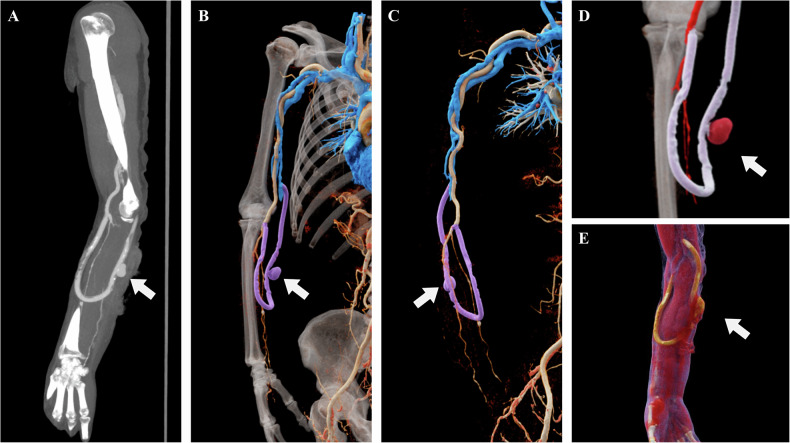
Follow-up images of a 65-year-old male after prosthetic arteriovenous fistula creation. A The CTA-based MIP image reveals an abnormal encapsulated hyperdense shadow surrounding the prosthetic graft, suggestive of hemorrhage (white arrow). B–D CR images stereoscopically and intuitively depict the morphology of the rupture site and hematoma, using anatomical segmentation and color coding to differentiate the artery, vein, prosthetic graft, and contrast extravasation following the rupture (white arrows). E The CR image further demonstrates the spatial and stratified relationships between the lesion (white arrow) and surrounding tissue structures, providing a precise anatomical roadmap for surgical exploration and repair
